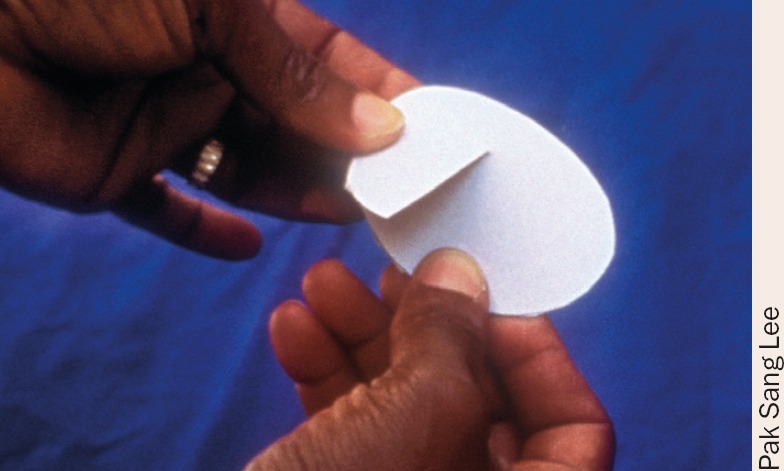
Extra protection can be given by taping a shield over the pad in the same way. The shield in Figure 6 is produced commercially and is called a Cartella shield. You can also make your own. Use a round object to draw a circle approximately 8 cm in diameter on thin cardboard ora used X-ray film and cut around it. Make a single cut into the centre (just half the diameter). Turn into a cone (Figure 7) and secure the shape with adhesive tape.
